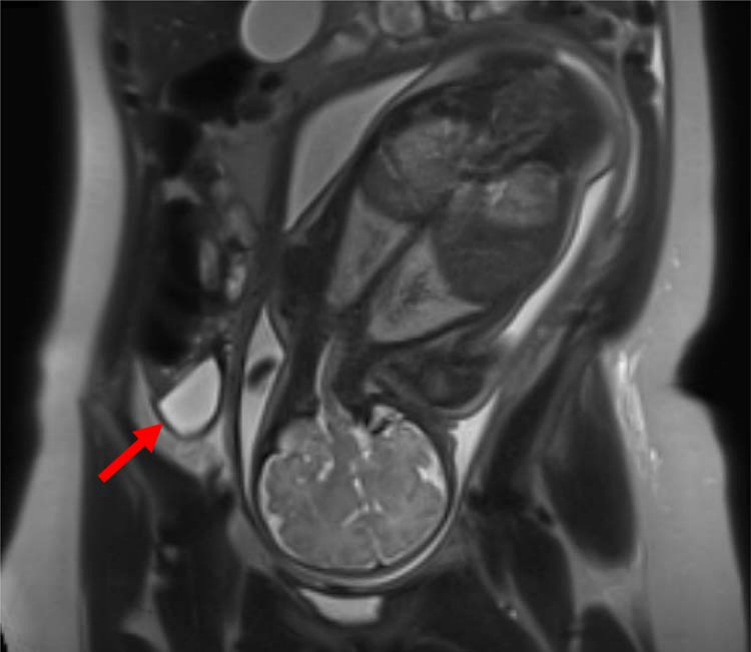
MRI of patient 1 revealing a fluid collection in the right lower abdomen.
